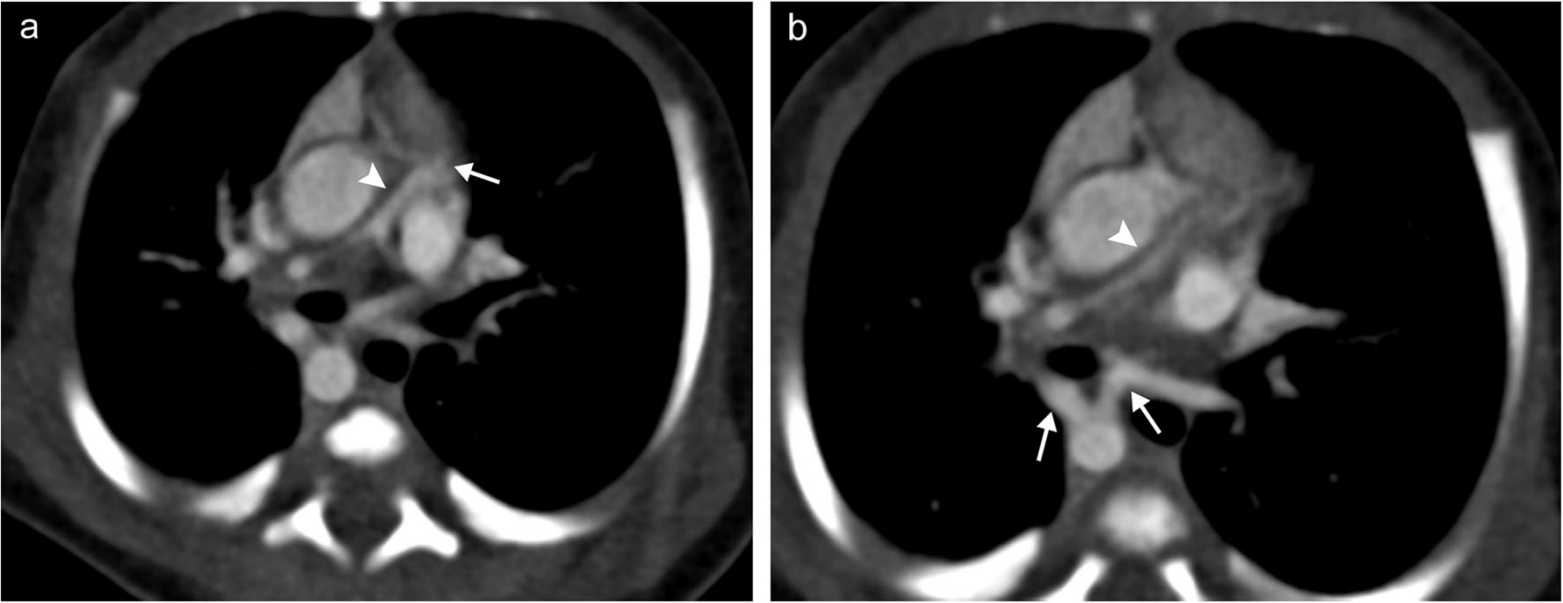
Pulmonary artery atresia. a Axial CT image demonstrating abrupt termination of the main pulmonary artery (*white arrow*) in a patient with tetralogy of Fallot with a small hypoplastic distal pulmonary artery (*white arrowhead*). b Bronchial artery dilatation off the descending aorta occurs to feed the pulmonary vasculature (*white arrows*) with the hypoplastic distal pulmonary artery again demonstrated (*white arrowhead*)
